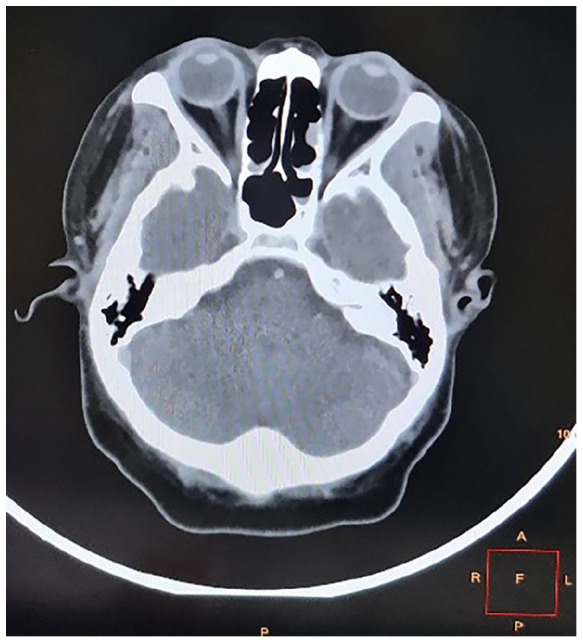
Axial section of brain. Figure 1 was found to have a focal irregular area of nodular non-enhancing soft tissue thickening in relation to the retina just adjacent to the optic nerve insertion, which appears hyperdense in the unenhanced study. This is suggestive of a retinal lesion (inflammatory, non-benign or haemorrhage) or artefactual. No calcifications are present. Intraocular and extracoronal spaces are intact bilaterally. Computed tomography (CT) image of patient’s brain.
